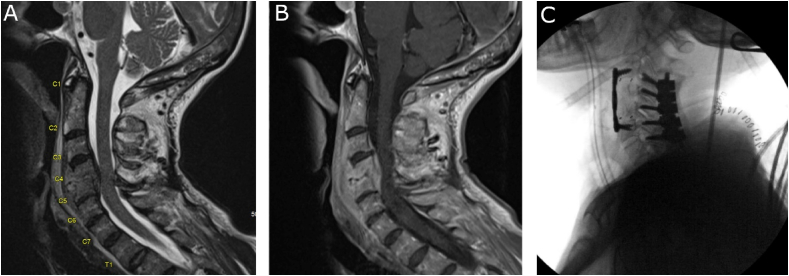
Example of a case of cervical spondylodiscitis with associated epidural abscess treated at our center. A: Sagittal T2 image demonstrating significant canal stenosis with developing kyphotic deformity centered at C4/5. B: Sagittal T1 post-contrast image demonstrating contrast enhancement within the disc and vertebral bodies of C4/5 with enhancing ventral epidural abscess from C3-7. C: Intra-operative lateral plain x-ray films demonstrating C3-7 posterior decompression/fusion with C4-5 corpectomy and C3-6 anterior decompression/fusion.
